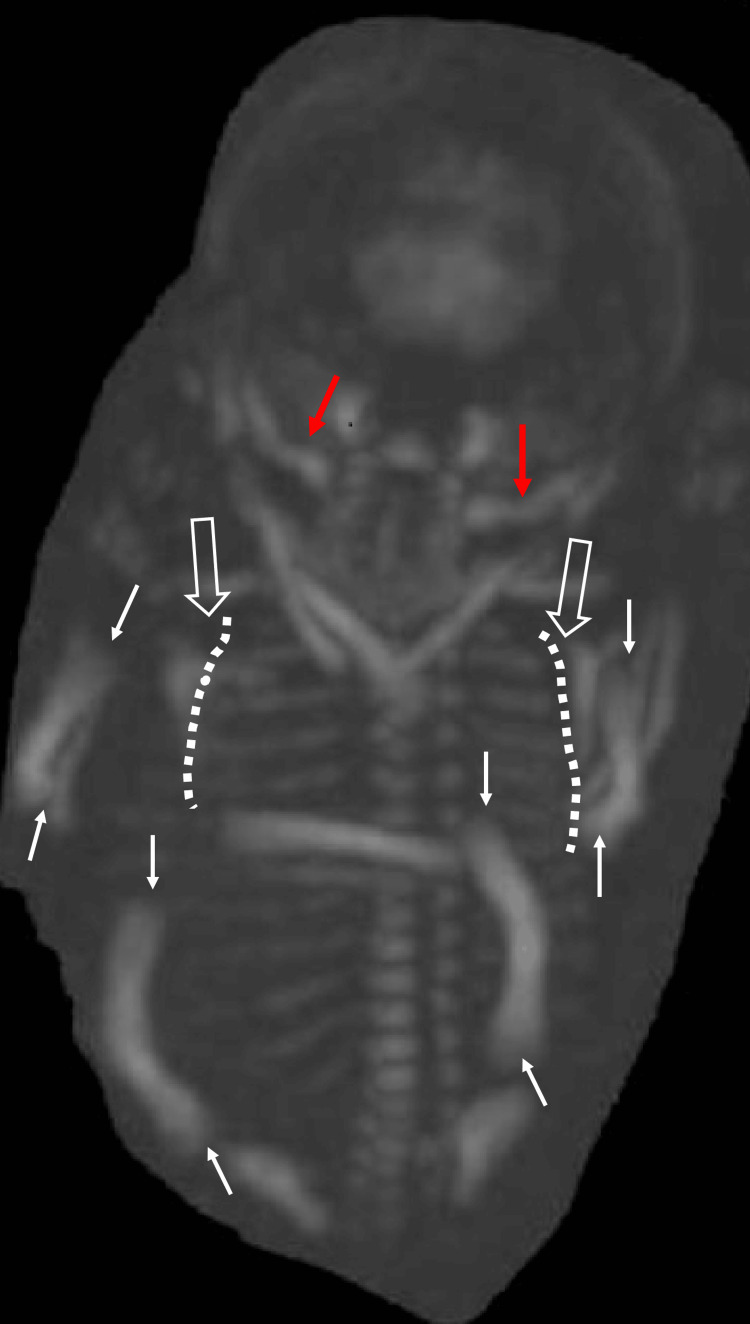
3D CT of the second pregnancy at 20 weeks and four days of gestation Fetal epiphyseal protrusion was cupping (white arrow), and the scapula was poorly detected (white open arrow), but thoracic hypoplasia (white dotted line) and periorbital ossification (red arrow) were noted. 3D CT: three-dimensional computed tomography
